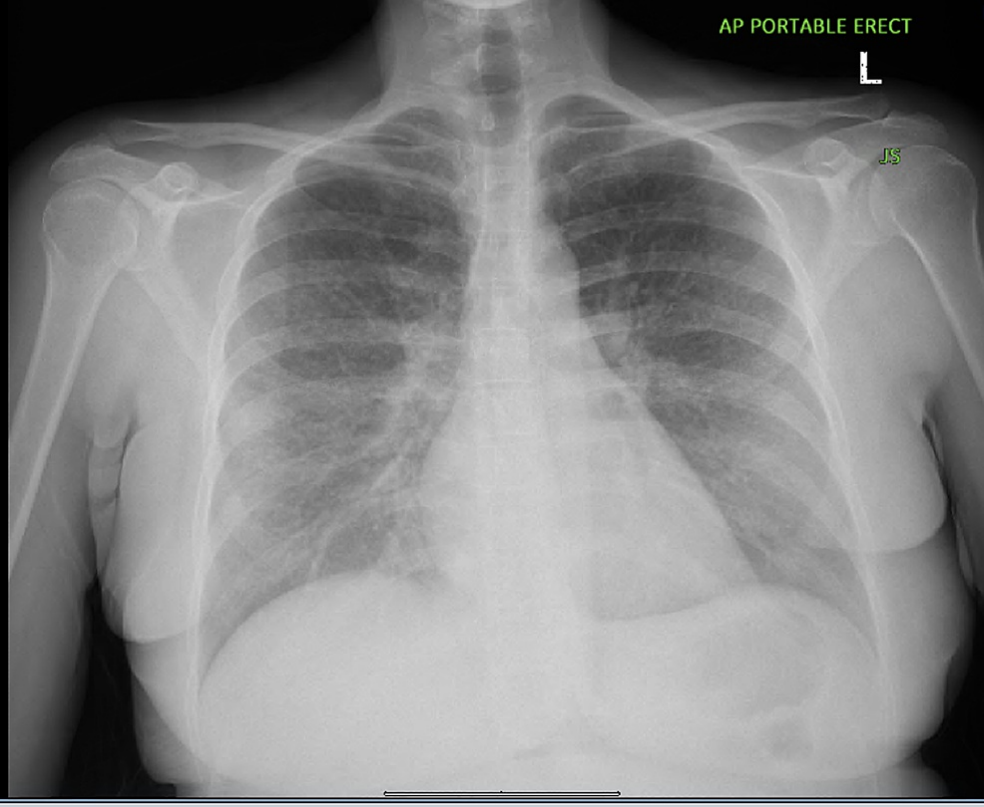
Chest X-Ray showing an ill-defined, generalized, hazy reticulonodular pattern of the lungs
